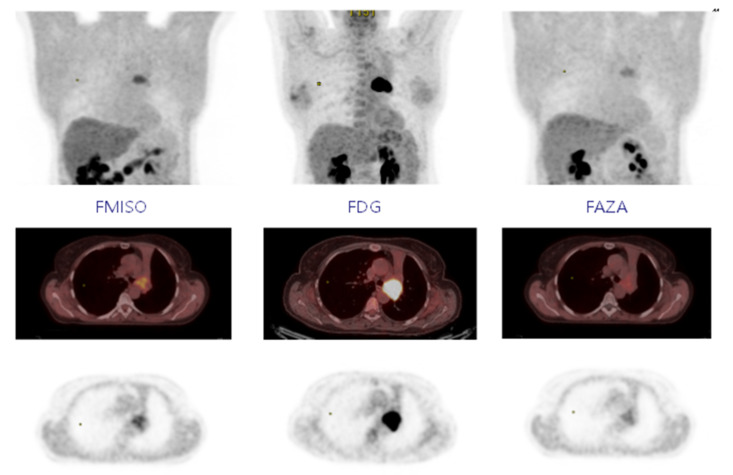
Fixing FMISO, FDG, and FAZA on MIP, axial PET-CT, and axial PET.
